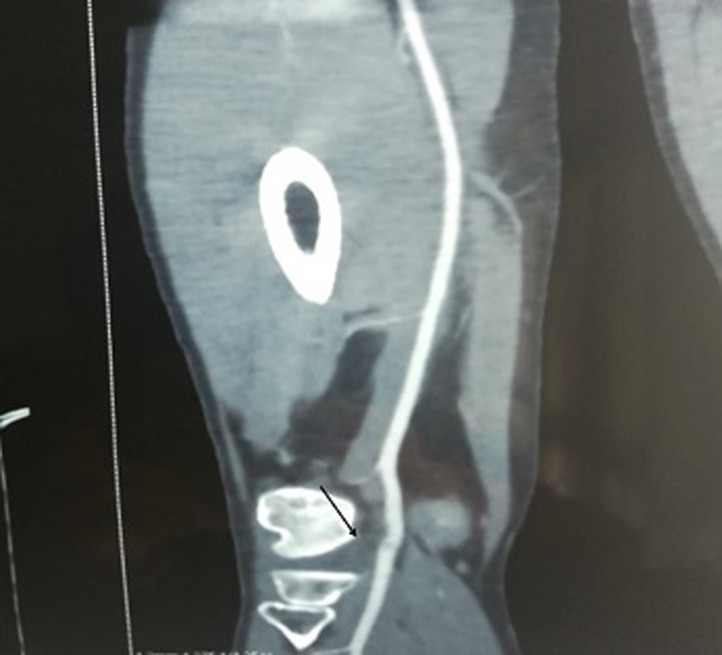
computerized tomography (CT) angiography showing the stenotic segment of the right popliteal artery (arrow)
